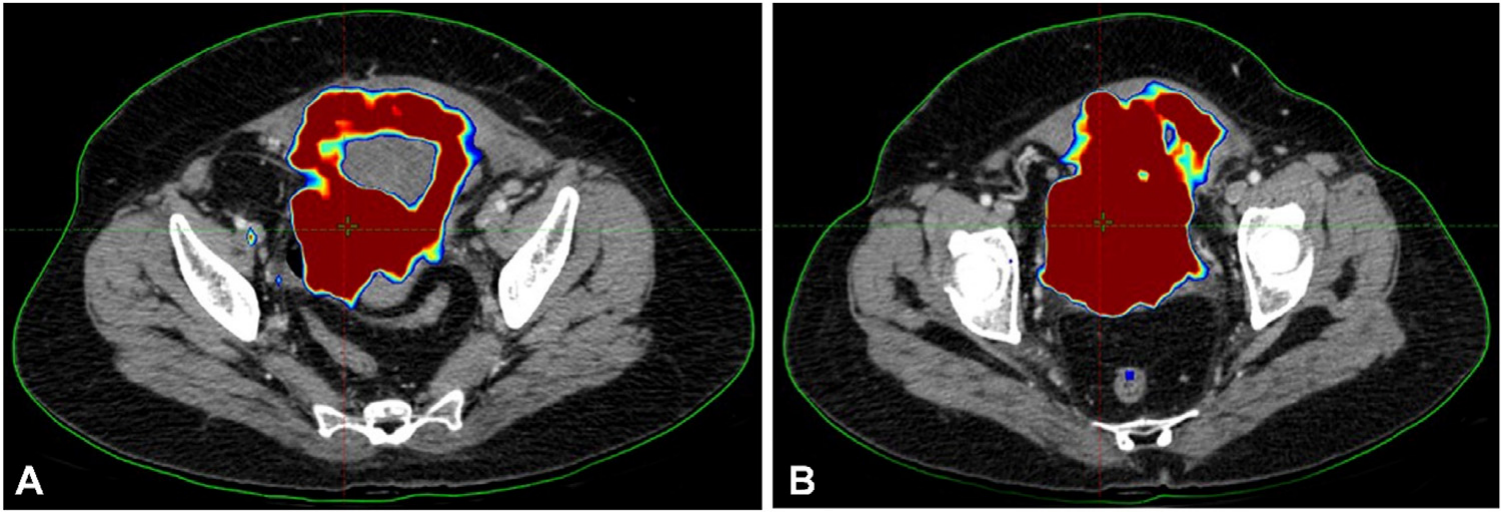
Radiation dose distribution planning on CT for bladder carcinoma.
